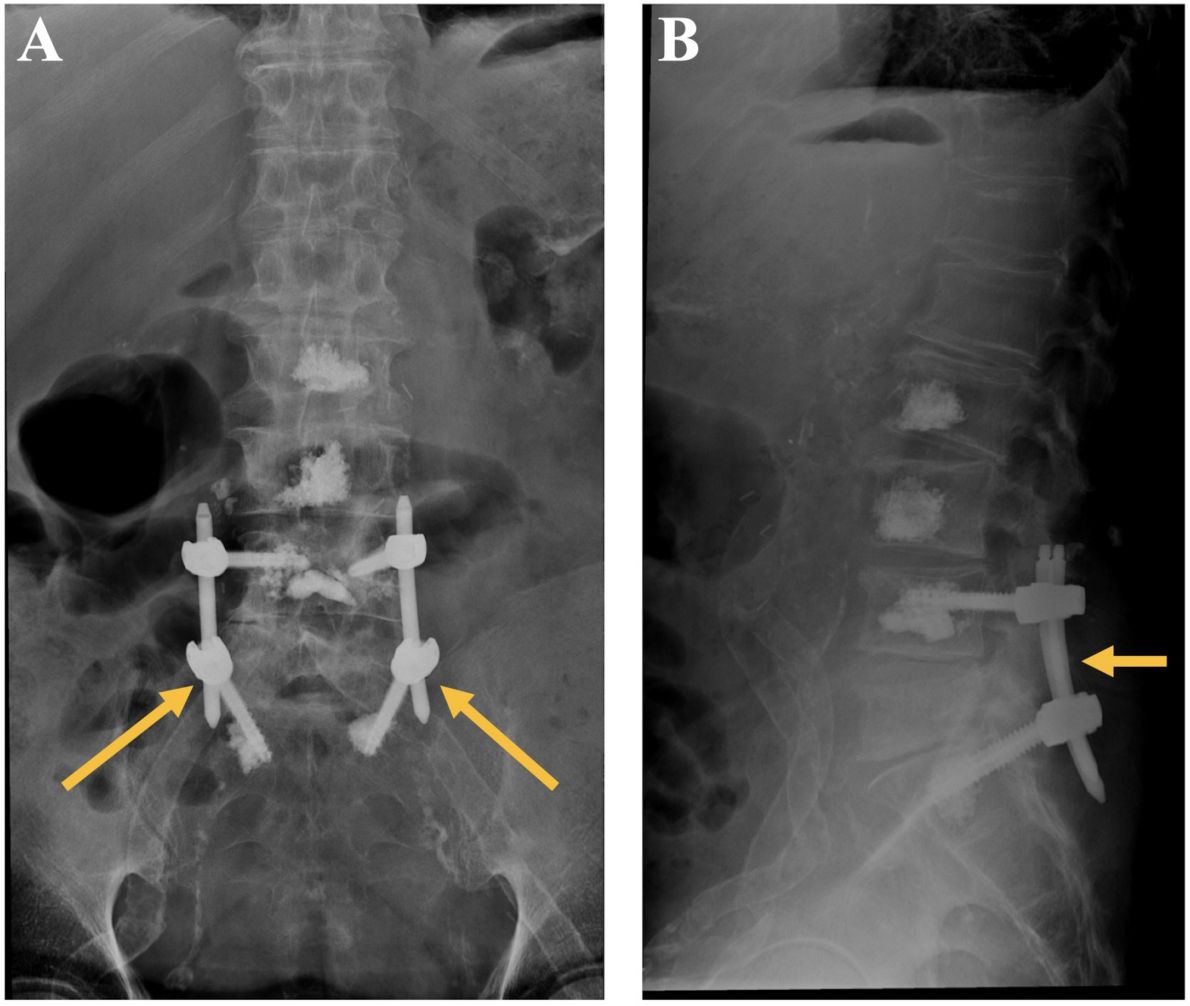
Repeat AP (A) and lateral (B) plain films of the lumbosacral spine two weeks post-operatively, demonstrating intact L4-S1 instrumentation, and early evidence of posterolateral bony fusion mass.
